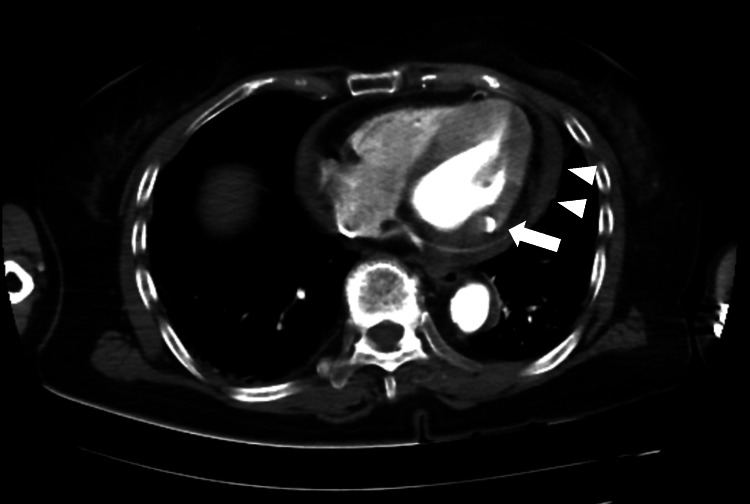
Contrast-enhanced computed tomography (CECT) imaging shows effusion from the left ventricle into the pericardial space (arrow) and pericardial effusion (arrowheads).
